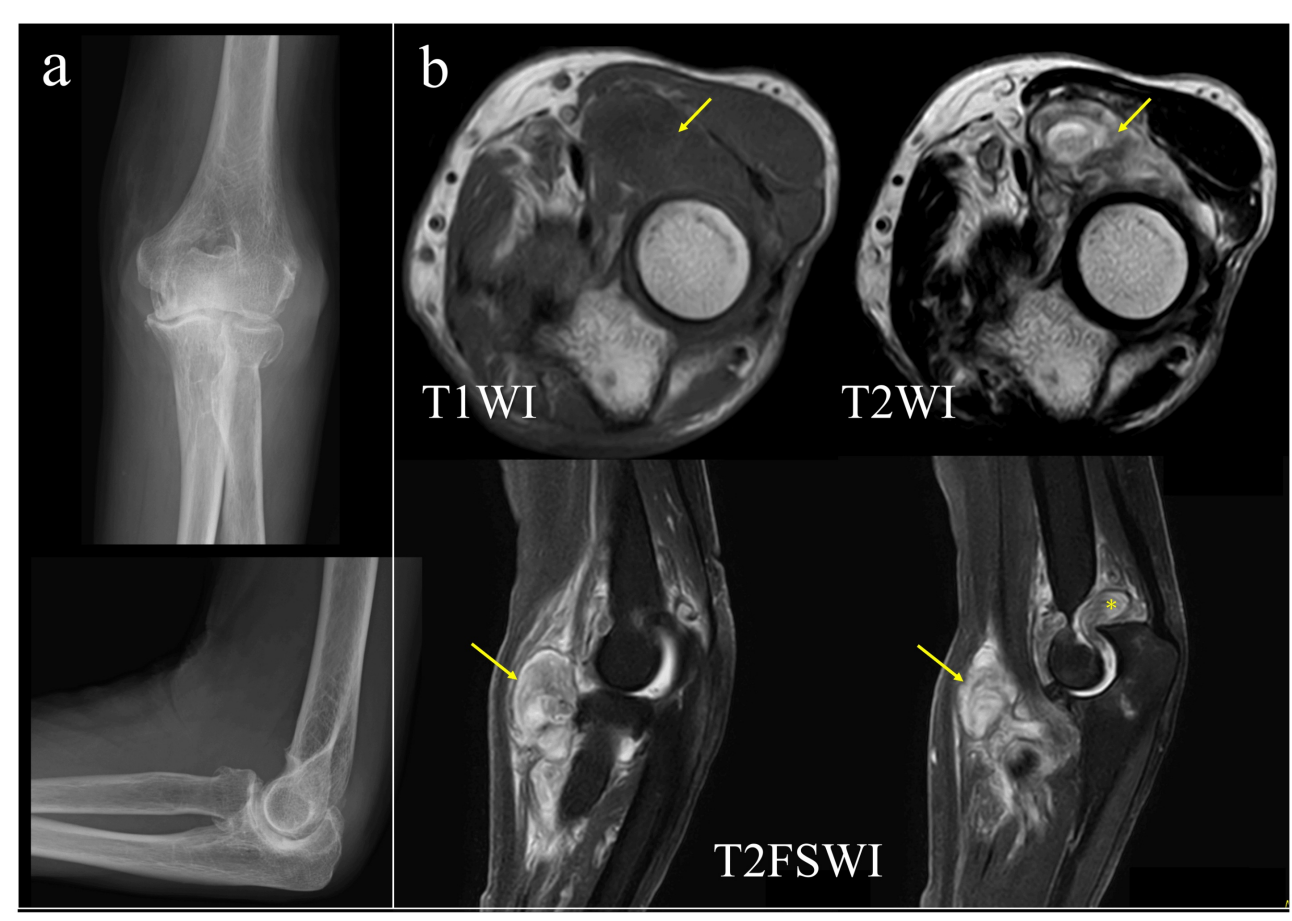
Initial imaging findings (a) Anteroposterior and lateral radiographs of the left elbow show mild joint space narrowing and bone erosion consistent with Larsen Grade 2. (b) MRI of the left elbow. Yellow arrows indicate the mass lesion, and (*) indicates enhanced synovial thickening in the elbow joint. MRI: Magnetic resonance imaging; T1WI: T1-weighted images; T2WI: T2-weighted images; T2FSWI: Fat-suppressed T2-weighted images
